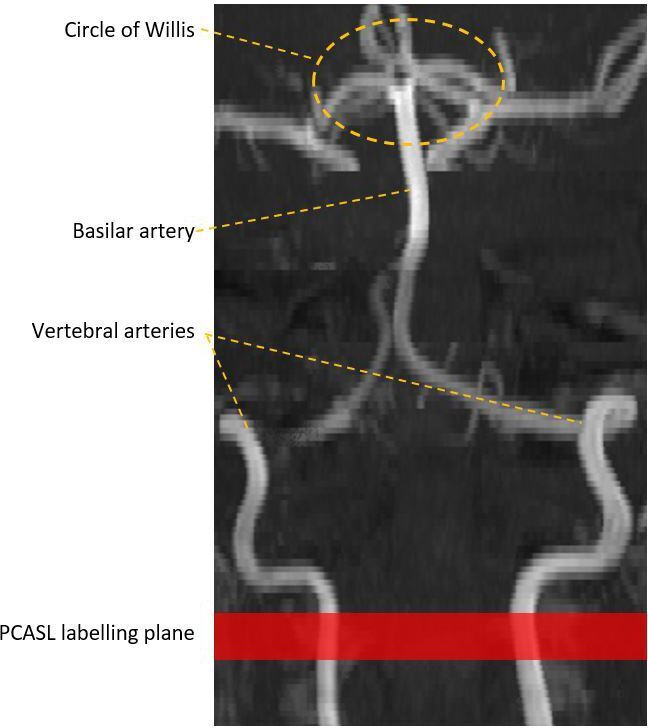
Time-of-flight MRI angiogram of the vertebrobasilar arteries demonstrating representative example of label location for brainstem ASL.
